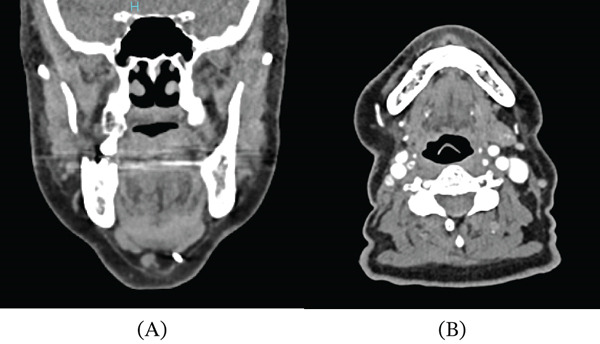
CT scan of the facial mass (January 15, 2025). (A) Coronal section and (B) axial section.
